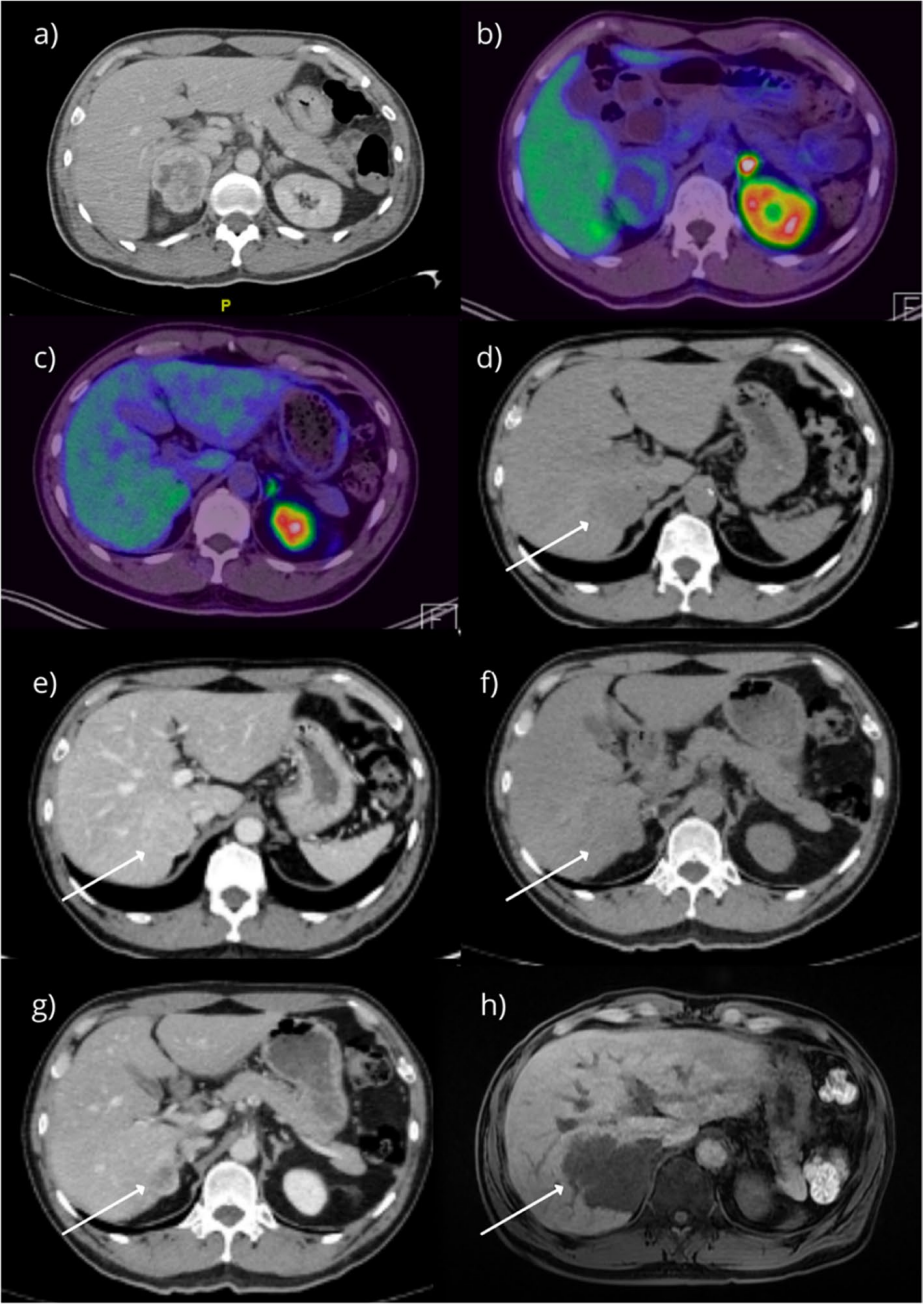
a CT Abdomen and pelvis, axial cut showing right adrenal lesion measuring 4.8 cm × 5.4 cm at index admission. b^68^Gallium-DOTANOC PET/CT showing DOTANOC avid right adrenal mass measuring 6.2 cm × 5.0 cm at index admission. c^68^Gallium-DOTANOC PET/CT showing DOTANOC avid right adrenal mass measuring 2.3 cm × 1.7 cm 2 years post-operatively. d Non-enhanced phase of adrenal CT showing mass measuring 3.2 cm × 2.9 cm (arrow) 2 years 6 months post-operatively. e Port-venous phase of adrenal CT showing mass measuring 3.2 cm × 2.9 cm with 77% absolute contrast washout 2 years 6 months post-operatively. f Non-enhanced phase of adrenal CT showing mass measuring 4.8 cm × 4.7 cm (arrow) 3 years after initial resection. g Porto-venous phase of adrenal CT showing mass (arrow) 3 years after initial resection. h MRI liver 3 years 6 months after initial resection showing mass measuring 5.2 cm × 6.7 cm × 6.2 cm in size with invasion into the right hepatic lobe involving segments 5–8 (arrow), areas of necrosis noted
